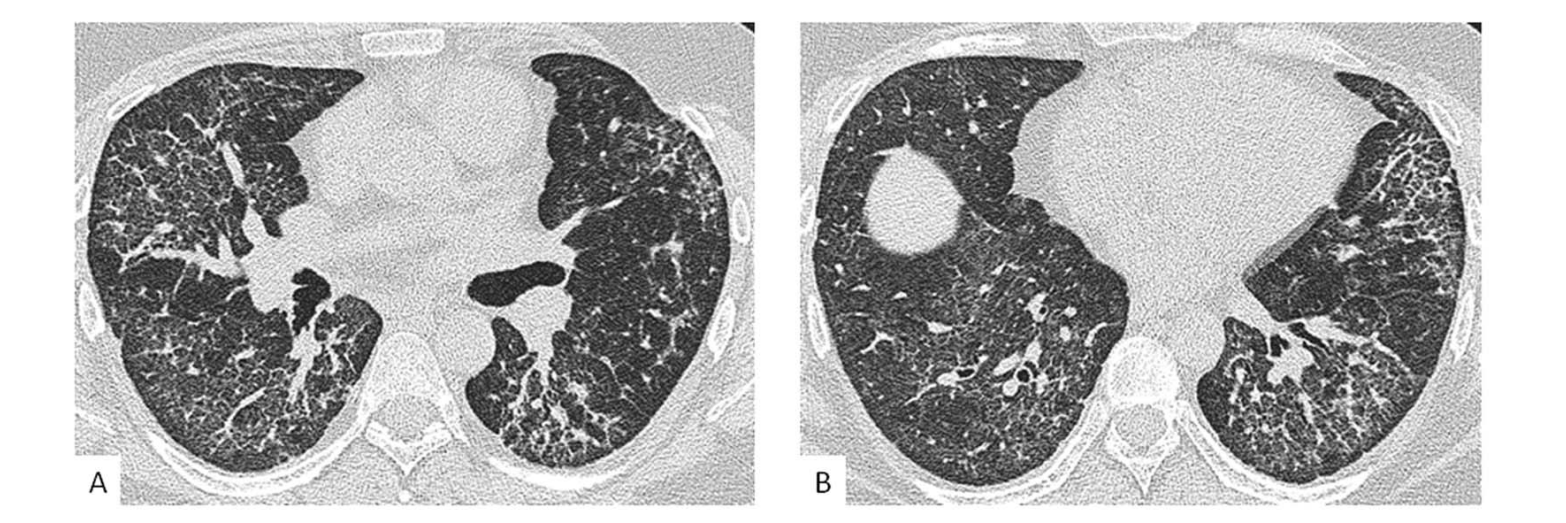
High resolution thoracic CT three years after diagnosis. High resolution CT sections at the level of a) the hilae and b) the lung bases demonstrate resolution of the previously noted consolidation and a reduction in ground glass attenuation. However evidence of fibrosis persists with bilateral reticular change and traction bronchiectasis. The dome of the right hemidiaphragm is visible in the image of the lung bases (b).
